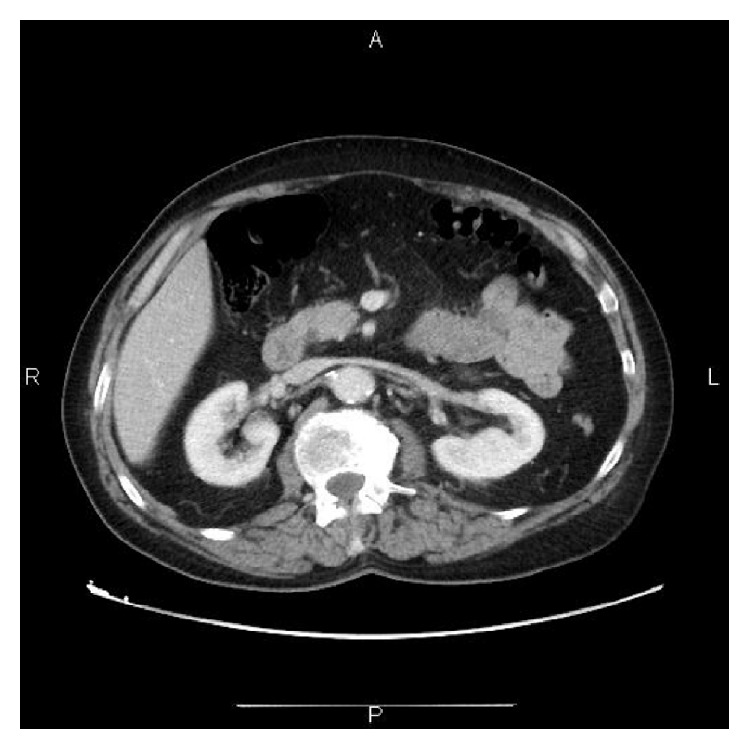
CT scan cross section: complete repermeabilisation of the inferior vena cava and of the renal veins.
